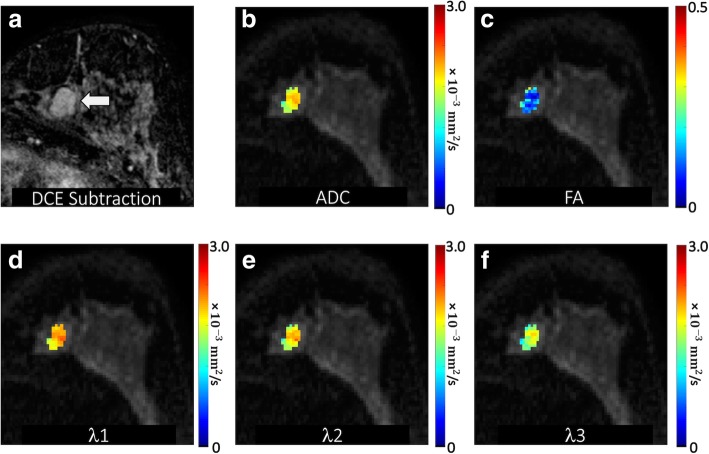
Benign mass detected in a 45-year-old patient undergoing MRI for high risk screening. a DCE post-contrast image demonstrates an 18-mm irregular mass with irregular margins in the left breast 7 o’clock (arrow), assigned a BI-RADS category 4. DTI-derived parametric maps of b apparent diffusion coefficient (ADC), c fractional anisotropy, and eigenvalues d
